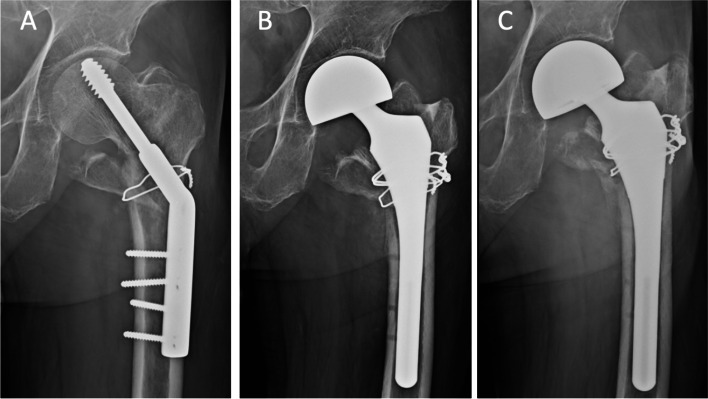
(A) 80-year-old male, with early fixation failure (postoperative 8 weeks, fracture collapse), (B) bipolar hemiarthroplasty with cementless primary stem, immediate postoperative radiograph, (C) stem subsidence at postoperative 6 months
